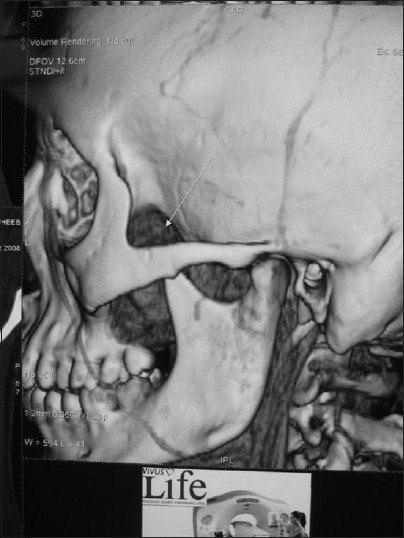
3-D Reconstruction CT scan is useful to assess involvement of bony structures, and for preoperative planning of the skull base tumor surgery
